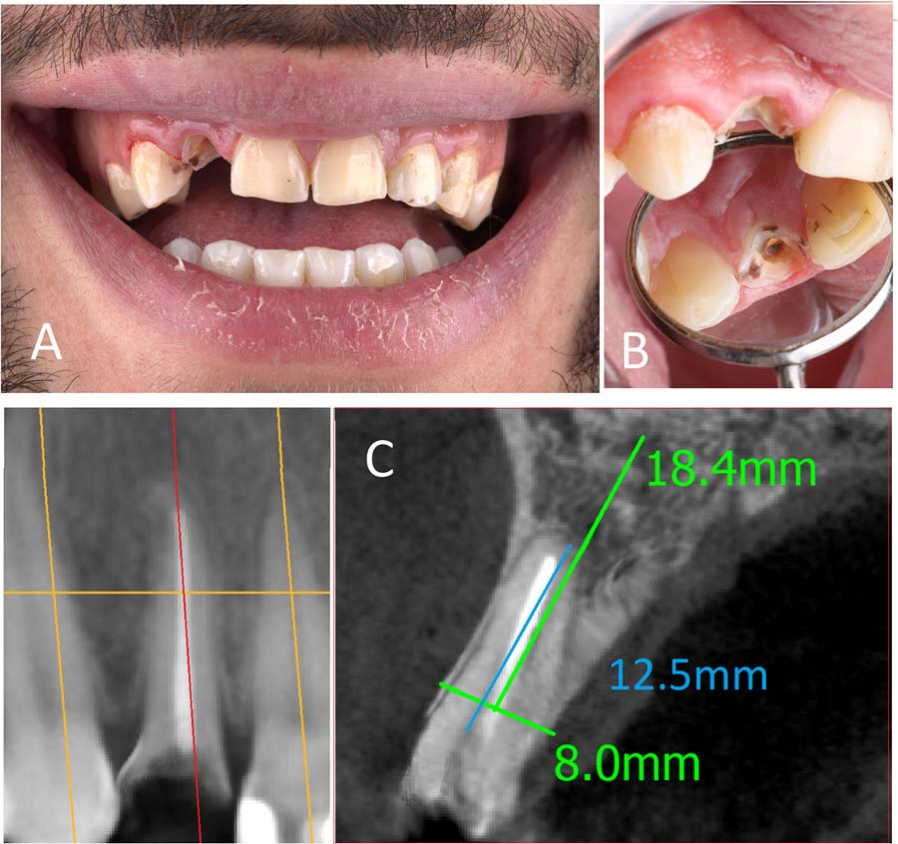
(A) Preoperative frontal view, (B) preoperative occlusal view, (C) preoperative CBCT.
